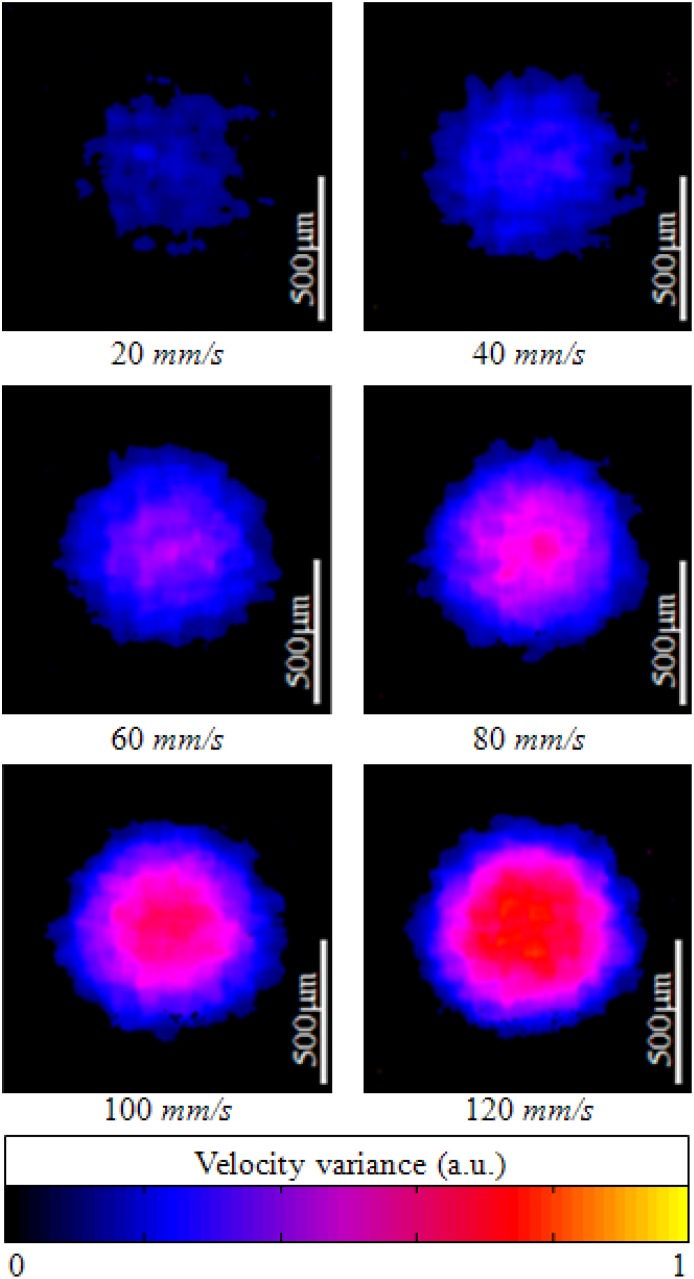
B-scan ODT images of capillary tube with different flow velocities. Doppler angle: 80°. Averaging window size: 12 + 20 (*M* + *N*).
